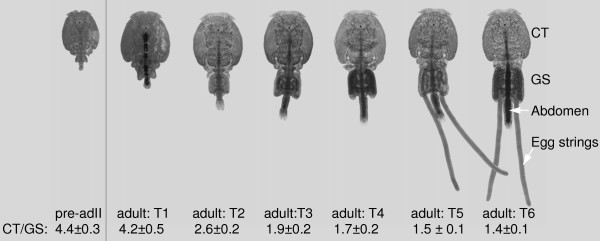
Post molting growth of adult female *L. salmonis*. The size of the cephalothorax (CT) is stable, while the genital segment (GS) and the abdomen (Ab) grow as indicated by the decreasing CT/GS ratio. The time frame from T1–T6 is approximately two weeks at 10°C. External egg strings may be observed approximately 10 days post molting.
